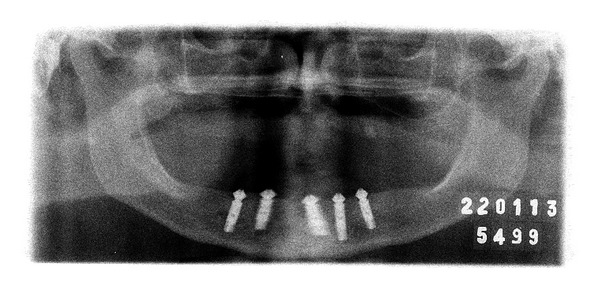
Radiographic control at 2-year follow-up.
